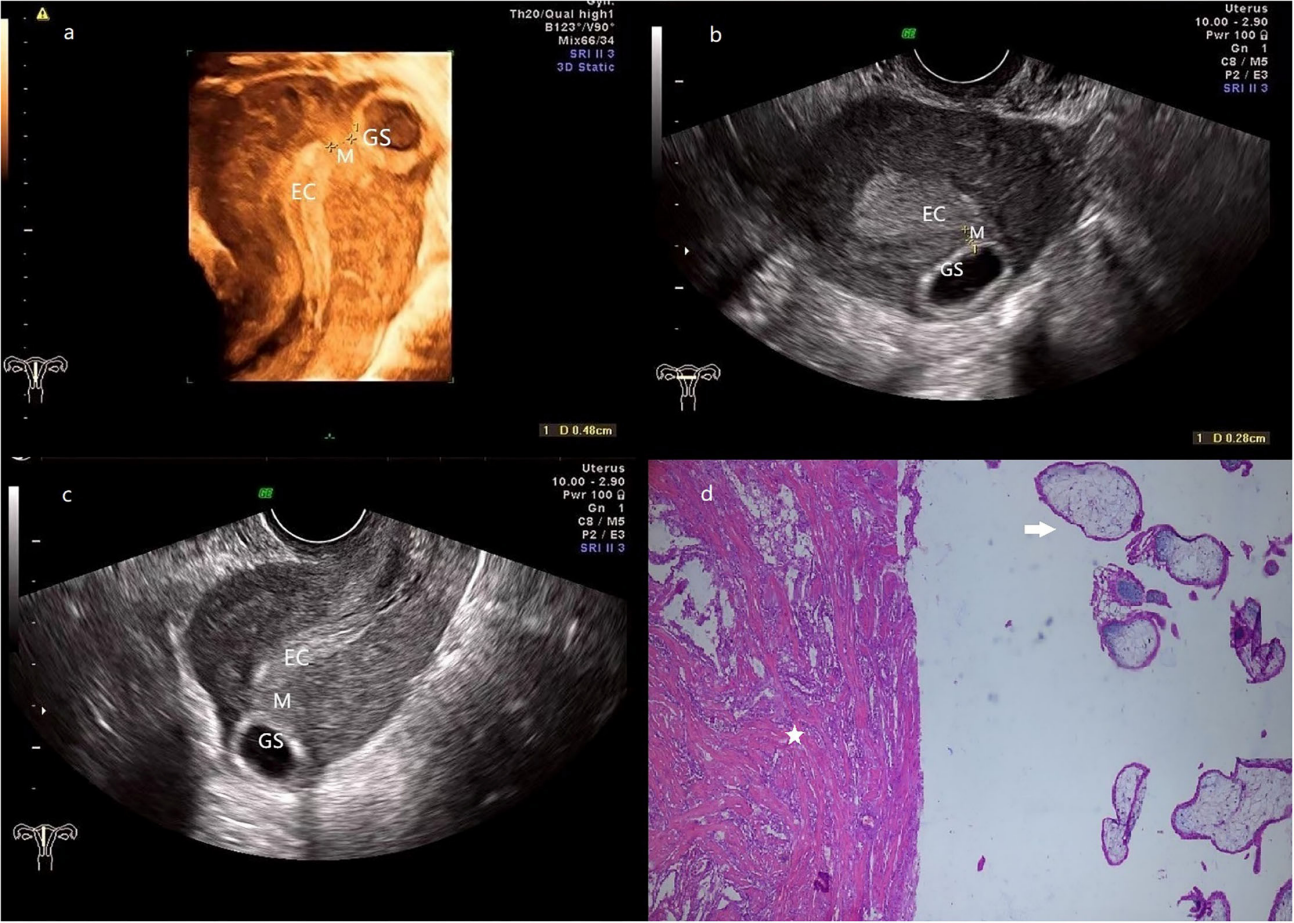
(a) 3-D ultrasonography reveals the relative location of EC, GS (a size of 2.7*1.0 cm), and the myometrium (0.5 cm in thickness) is located between them. (b,c) transversal (b) and sagittal (c) section image showed that the GS is located at the left posterior wall of the fundus, and the connection with EC is disrupted by the myometrium. (d) The pathologic examination demonstrates that muscular tissue (star) coexists with the chorionic villus (arrow). (Patient 2, GS, gestational sac; M, myometrium; EC, endometrial cavity).
